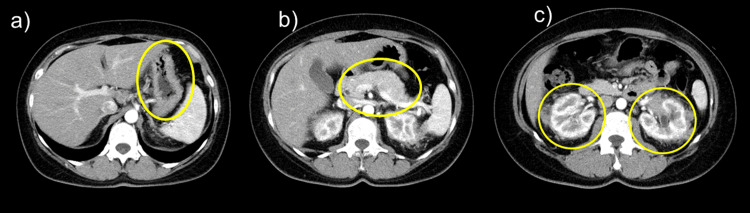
Contrast-enhanced CT of the chest and abdomen CT shows mild thickening of the gastric wall (a) and slight enlargement of the pancreas without a capsule-like rim (b). The kidneys exhibit mild contour irregularity with increased density of the surrounding fat and thickened fascia (c) (yellow circles). CT, computed tomography
